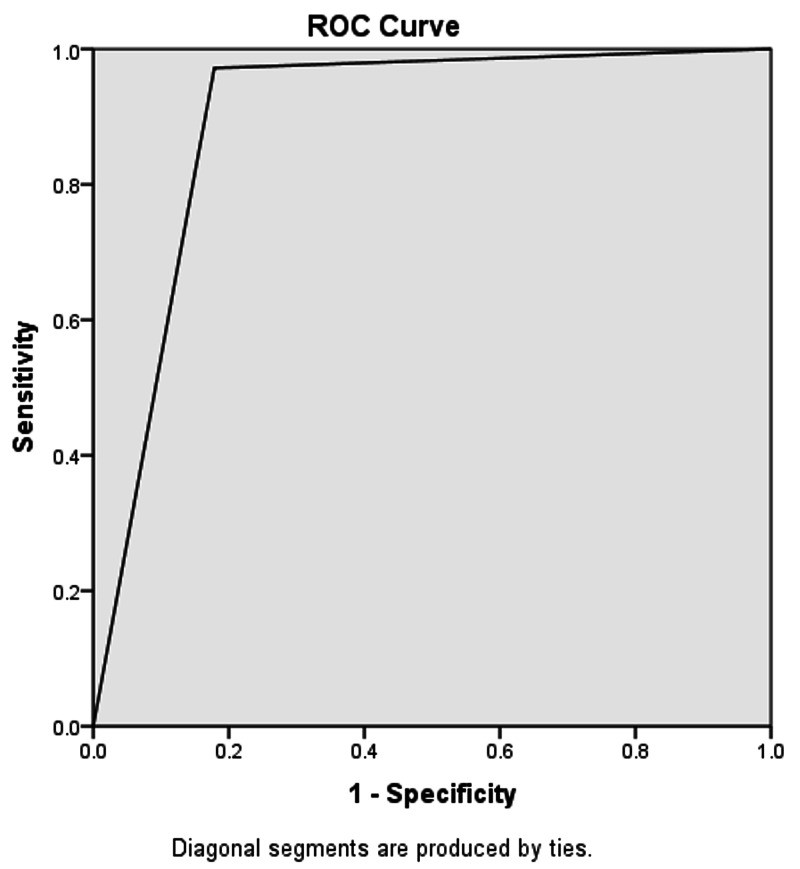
Area under the curve for combined TLC, neutrophil count, and U/S = 0.897 TLC: total leukocyte count; U/S: ultrasound
